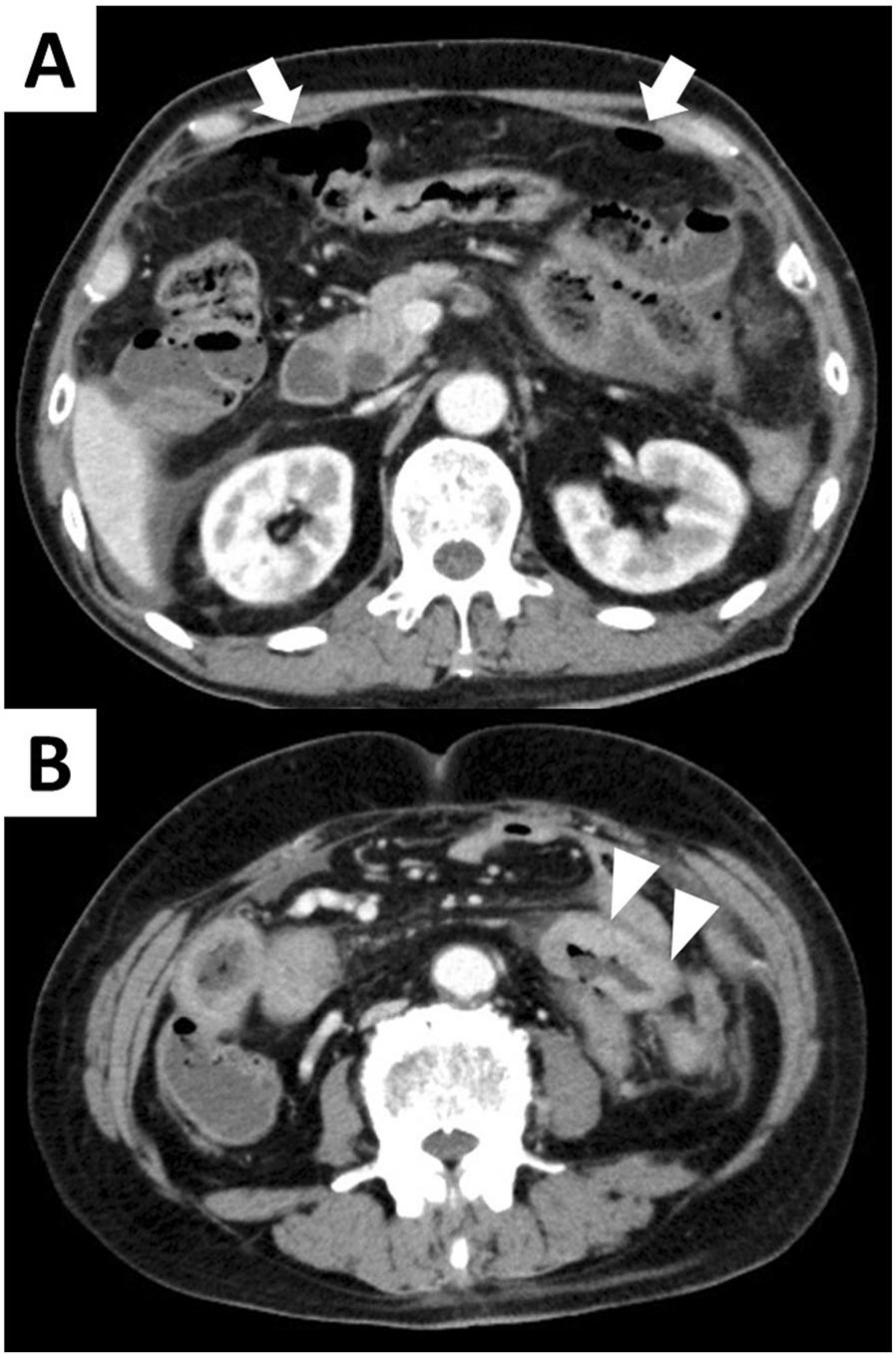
a, b Abdominal enhanced CT pictures are shown. Arrows show abdominal free air, and triangles show the perforation site of small intestinal metastasis of lung adenocarcinoma
